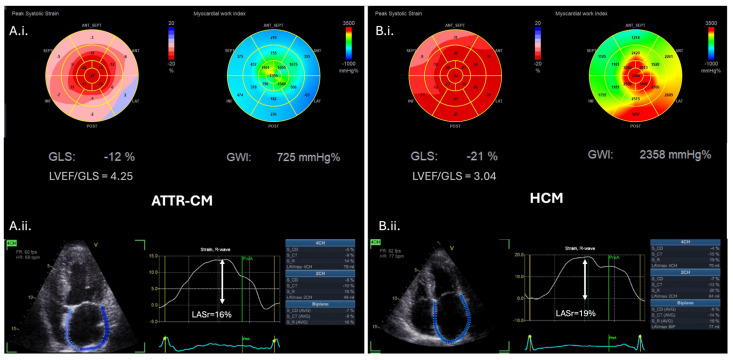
Advanced echocardiographic red flags in ATTR-CM (A.i.,A.ii.) versus hypertrophic cardiomyopathy (HCM) patients (B.i.,B.ii.). (A.i.) Bull’s eye plots of left ventricular global longitudinal strain (GLS) and myocardial work index (MWI) in an ATTR-CM individual demonstrating an apical sparing pattern versus (B.i.) the respective Bull’s eye plots of a HCM individual demonstrating reduced left ventricular GLS and MWI of the hypertrophic interventricular septum. (A.i.) and (B.i.) LVEF/GLS ratio is higher in CA compared to HCM (cut-off value LVEF/GLS ratio > 4.1 to diagnose CA with 89% sensitivity and 91% specificity) [55]. (A.ii.) Left atrial strain (LAS) analysis of the ATTR-CM patient versus (B.ii.) the respective LAS analysis of the HCM patient, indicating the lower peak values of left atrial reservoir (LASr) and contractile strain characterizing ATTR-CM. ATTR-CM: transthyretin amyloid cardiomyopathy, CA: cardiac amyloidosis, GLS: global longitudinal strain, HCM: hypertrophic cardiomyopathy, LAS: left atrial strain, LASr: left atrial reservoir strain, LVEF: left ventricular ejection fraction, and MWI: myocardial work index.
